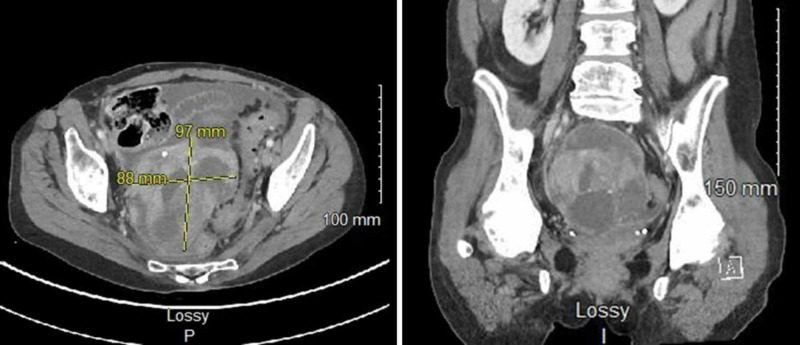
CT scan for abdomen and pelvis with contrast shows a large pelvic mass measuring 9.7 cm x 8.8 cm on the cross-sectional image (left), the coronal section showing lesional punctate hyperdensities compatible with ossification (right).
